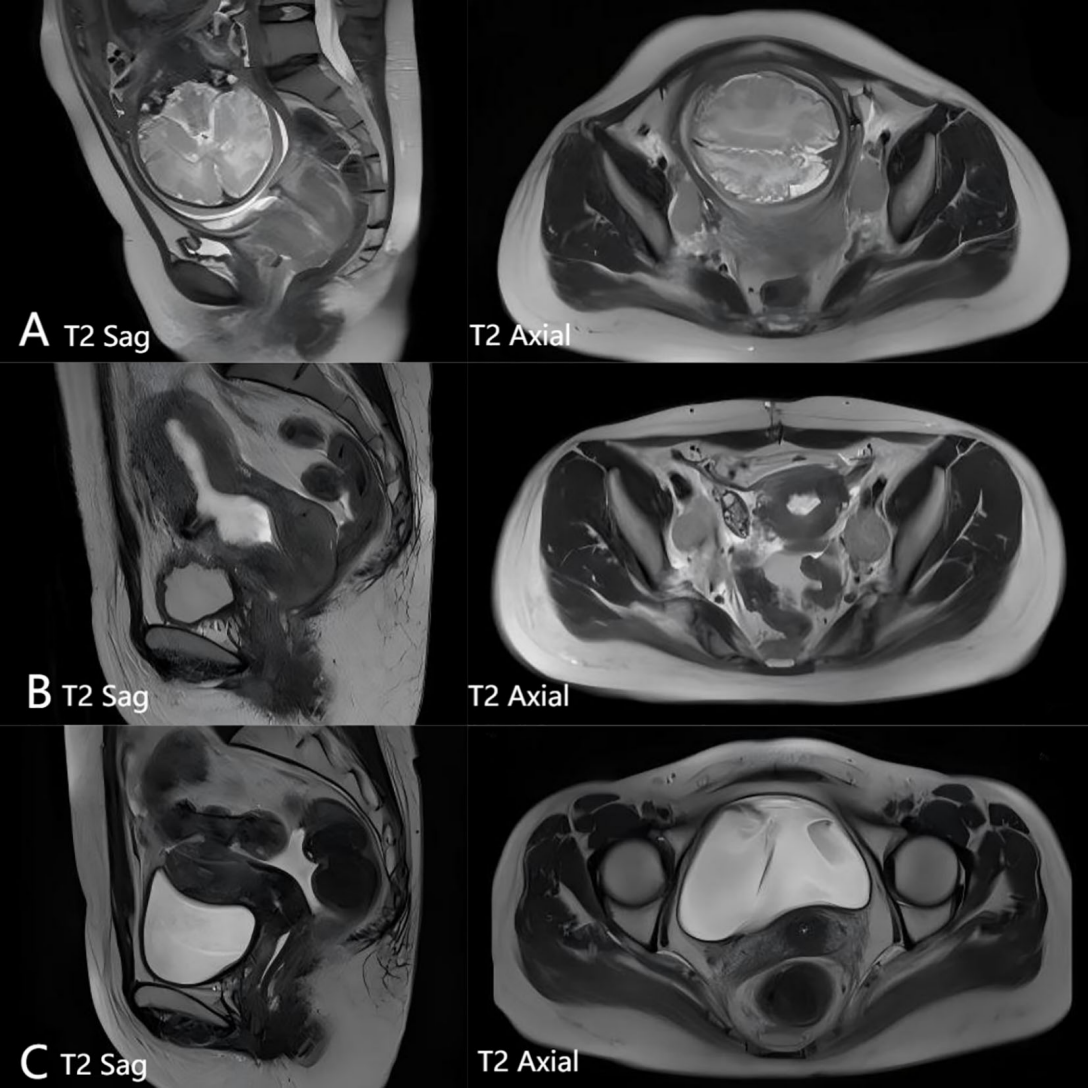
(A) Pelvic magnetic examination before treatment: Cervical mass with maximum transverse dimension of 7.9 cm × 5.6 cm and longitudinal dimension of 4.2 cm. Bilateral parametrial fat planes involved. Vaginal vault involved. No significant involvement of bladder or rectum noted. Multiple enlarged lymph nodes were noted bilaterally along the internal and external iliac vessels, with the largest located on the left side, measuring approximately 3.4 cm × 2.5 cm. Single intrauterine pregnancy, cephalic presentation, with the umbilical cord wrapped twice around the neck. (B) 2 PE cheng solution after chemotherapy pelvic MRI examination: Cervical mass significantly reduced in size compared to previous imaging, with maximum transverse dimension of 4.1 cm × 3.4 cm and longest diameter of 3.7 cm. Bilateral parametrial fat spaces involved, vaginal vault involved. No significant involvement of bladder or rectum noted. Multiple enlarged lymph nodes were observed along the internal and external iliac vessels bilaterally. The largest node, located on the left side, measured approximately 2.7 cm × 2.8 cm × 4.8 cm and showed reduction in size compared to previous findings. (C) Pelvic MRI check following treatment: Cervical lesion has almost disappeared.
